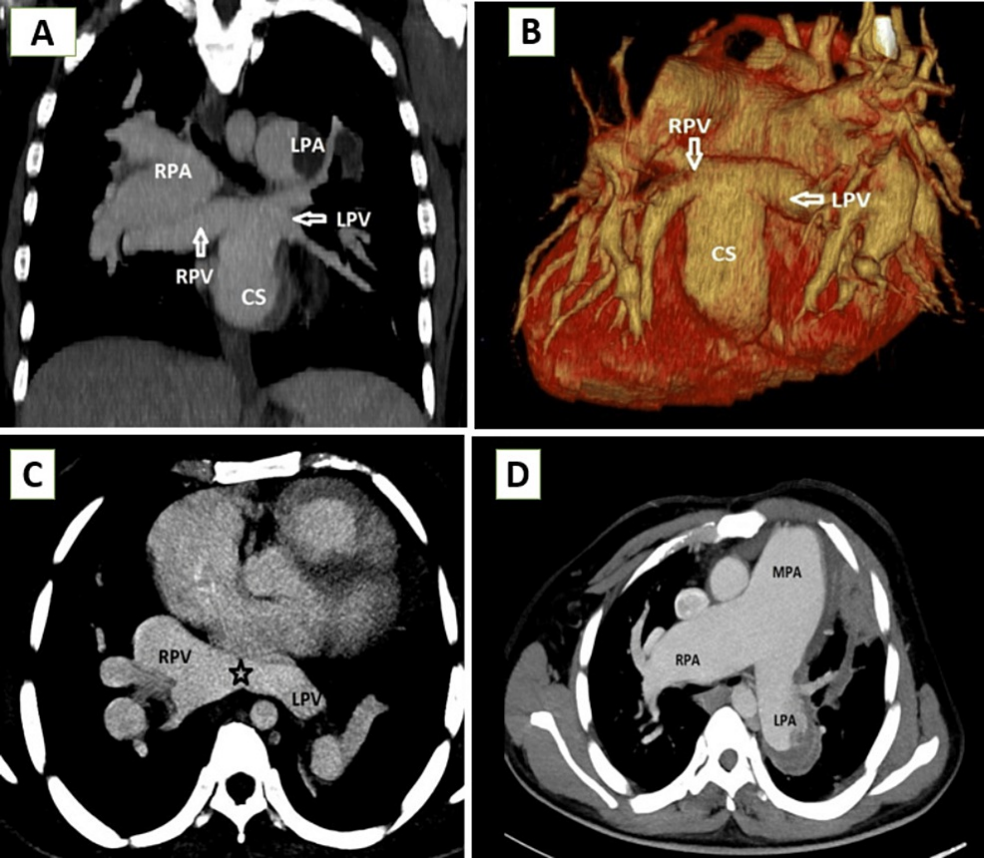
Intracardiac TAPVC (A and B) CT pulmonary angiography coronal MIP section (A) and 3D VR (B) images showing confluence of all four pulmonary veins draining into the dilated CS, (C) post-contrast axial MIP image showing confluence (black star) of all four pulmonary veins, and (D) post-contrast axial MIP image showing dilated MPA, RPA, and LPA and chronic thromboembolism involving the LPA and its upper lobar branches with infarct in the lingula TAPVC: total anomalous pulmonary venous connection, MIP: maximum intensity projection, VR: volume rendered, CS: coronary sinus, MPA: main pulmonary artery, RPA: right pulmonary artery, LPA: left pulmonary artery, RPV: right pulmonary vein, LPV: left pulmonary vein
